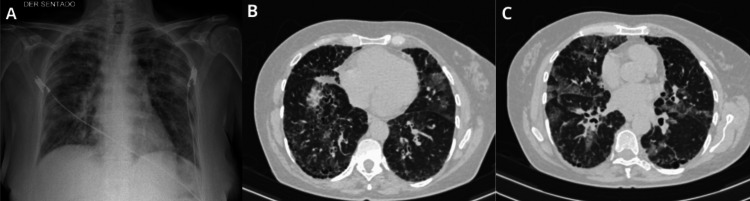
Diagnostic imaging. (A) AP chest radiograph showing bilateral interstitial opacities.
